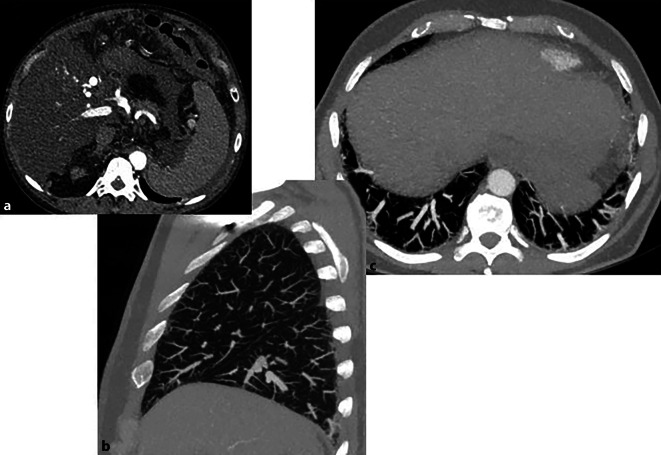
Hepatopulmonales Syndrom bei Leberzirrhose. a Axiale CT-Abdomenaufnahme: nodulär-zirrhotischer Umbau des Leberparenchyms sowie eine im Rahmen der portalen Hypertension begleitende Splenomegalie. b Axiale CT-Thorax: Zeichen eines hepatopulmonalen Syndroms mit bilateraler Dilatation peripher-subpleuraler Pulmonalarterienäste sowie einer vermehrten Anzahl terminaler Gefäßverzweigungen bis zur Pleura. c Sagittale Maximumintensitätsprojektions(MIP)-Rekonstruktion bestätigt die Ausdehnung der pulmonal-arteriellen Gefäßäste bis unmittelbar an die Pleuragrenze
